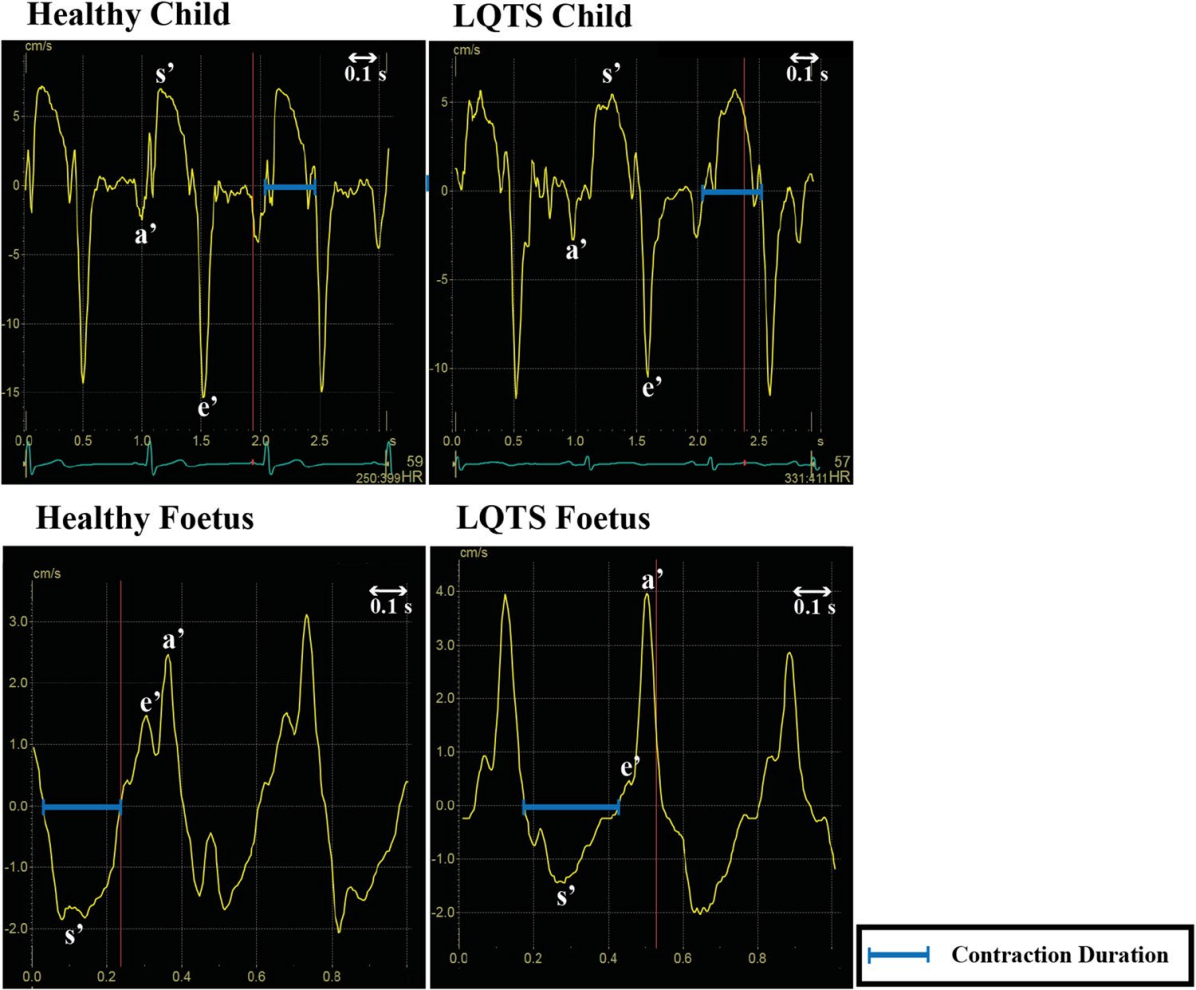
Myocardial contraction duration by cTDI in children (both upper panels) and in foetuses (both lower panels). Note that in the two lower panels the configuration of the velocity curves is inversed compared to the curves in children, due to a different position of the foetal heart with respect to the probe of the echocardiogram
